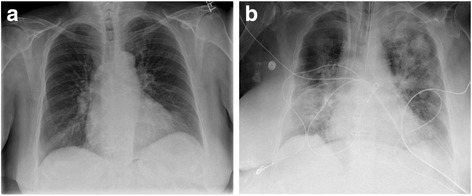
Pre-transfusion a and post-transfusion b chest x-rays
